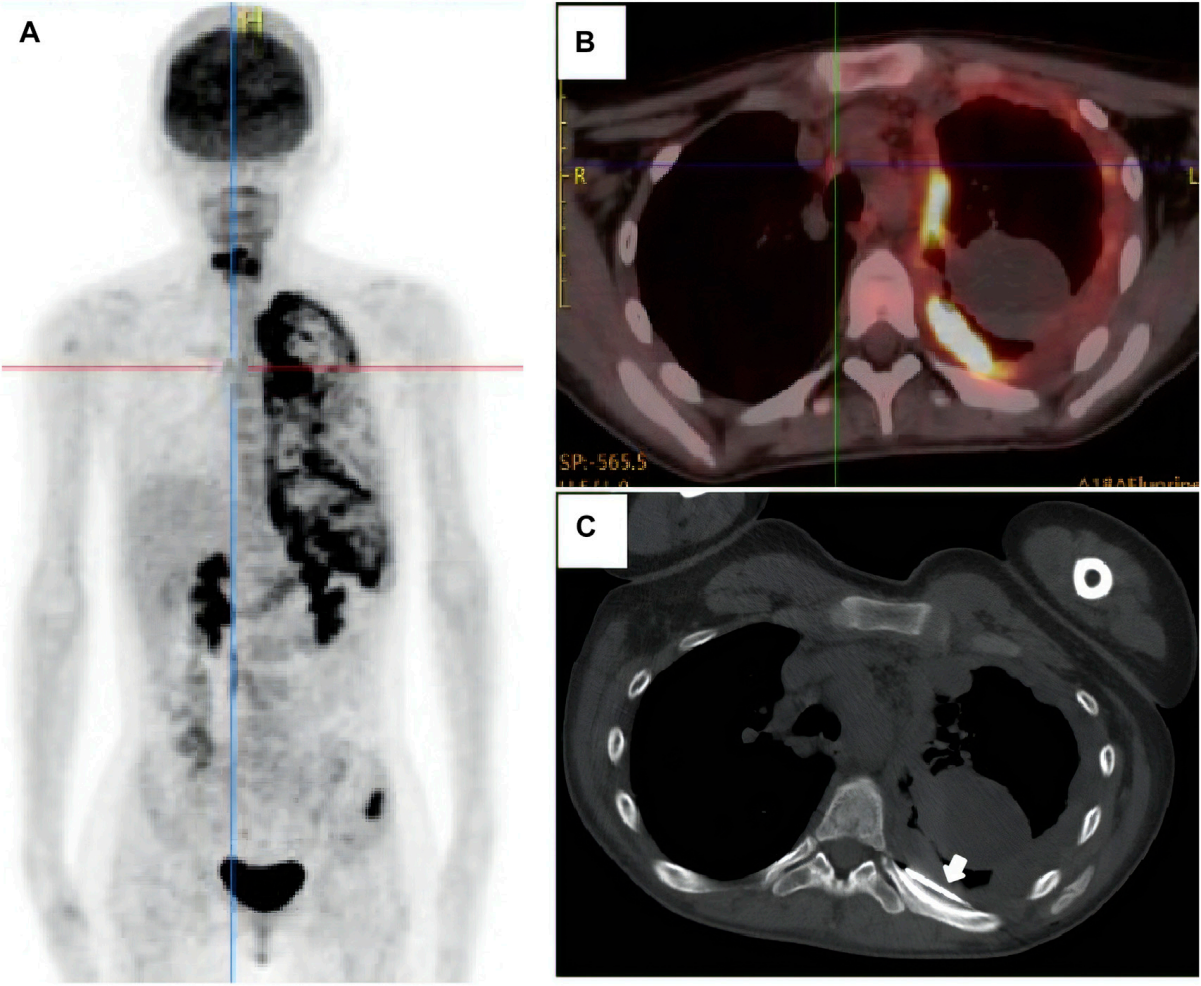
The positron emission tomography/computed tomography scan showed increased metabolic activity in the left iliac bone, the 6th cervical vertebra and the whole left pleura (A, B). The patient underwent CT-guided biopsy of the left pleural mass [(C), arrow].
